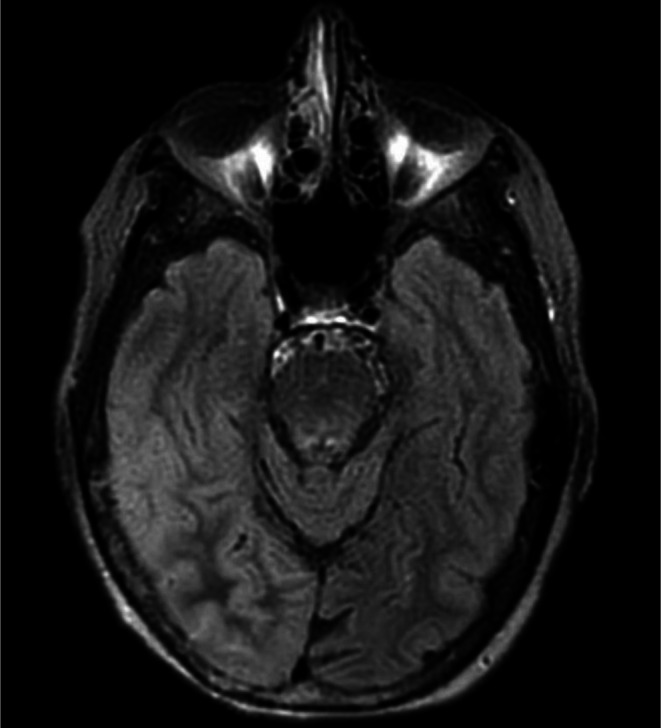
MRI brain (T2‐FLAIR) shows mild signal hyperintensity in the right parietal, occipital, and temporal regions compared to prior imaging, with sulcal effacement and mild mass effect.
